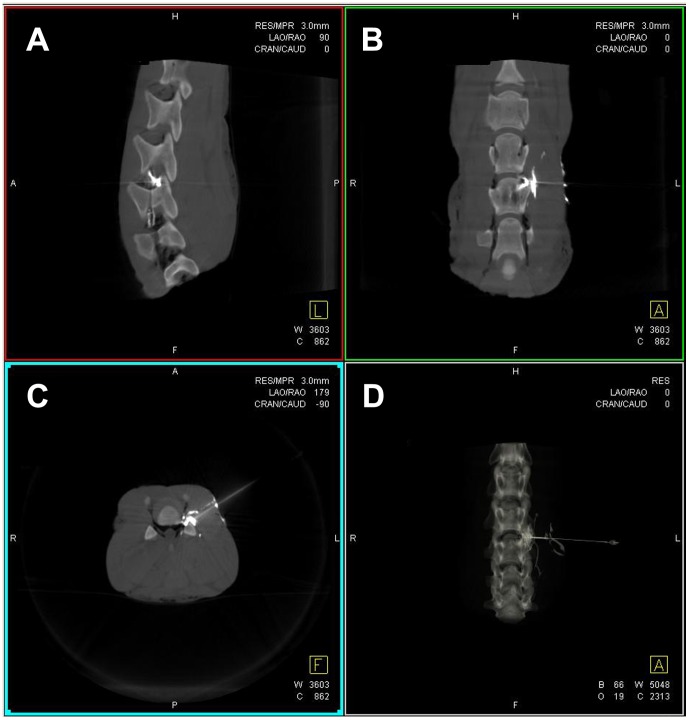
Contrast dye distribution in post-injection control Dyna-CT images. Lateral (A), coronal (B), and axial views (C) of the multiplanar reconstructions, as well as (D) three-dimensional rendering of the Dyna-CT of the *ex-vivo* lamb spine-model demonstrating correct distribution of the contrast material within the neural foramen after contrast dye injection.
